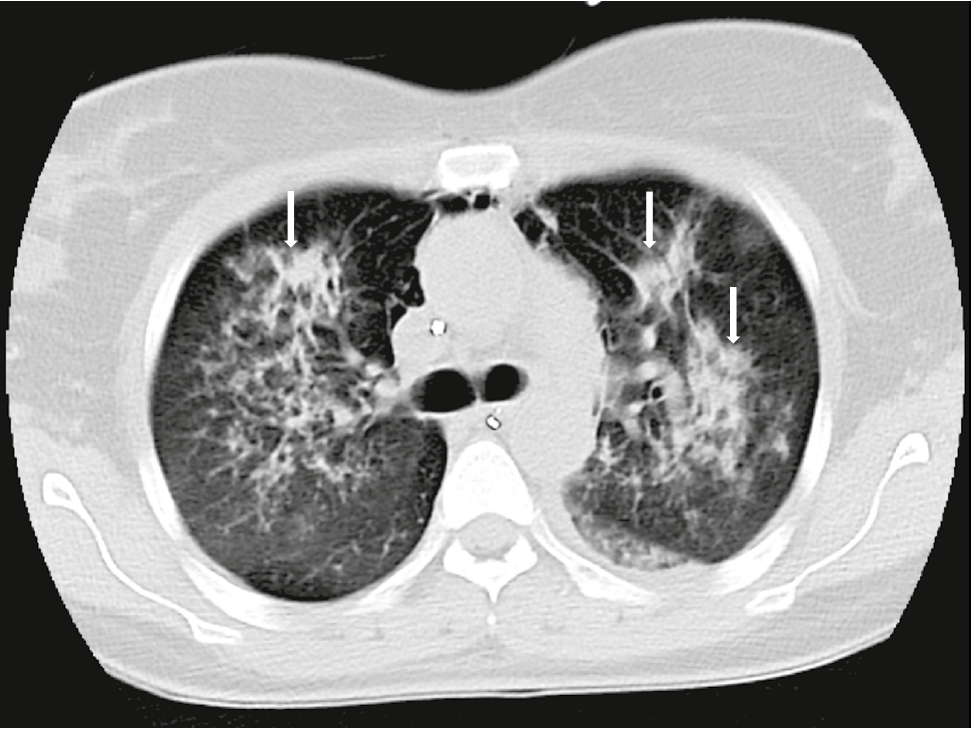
CT scan showing extensive bilateral consolidations in the lungs (Case 2)
